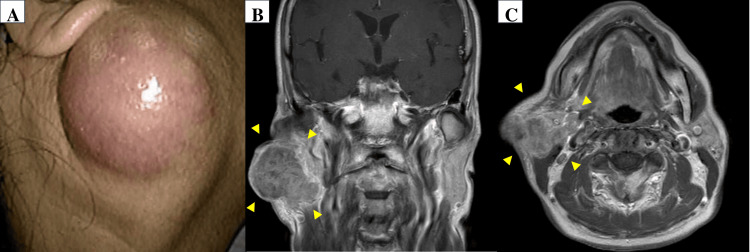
Right parotid tumor and enlarged level II lymph nodes (A) A mass lesion, approximately 45 mm in size, was identified within the right parotid gland. (B, C) Contrast-enhanced MRI of the head and neck showed a 45 mm × 38 mm tumor within the right parotid gland, demonstrating partial contrast enhancement and subcutaneous invasion; the parotid gland appeared fused with enlarged level II lymph nodes.
